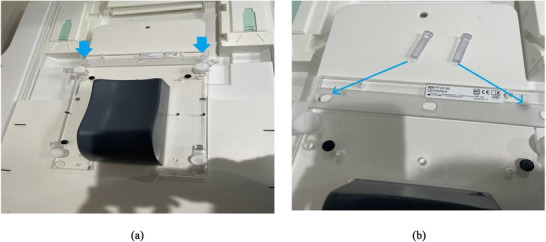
MR sim custom head rest jig with blue arrows pointing to the location of pegs connecting the jig to the MRI table with a full view of the jig (a) and zoomed in view (b) of the jig showing the custom pegs.
